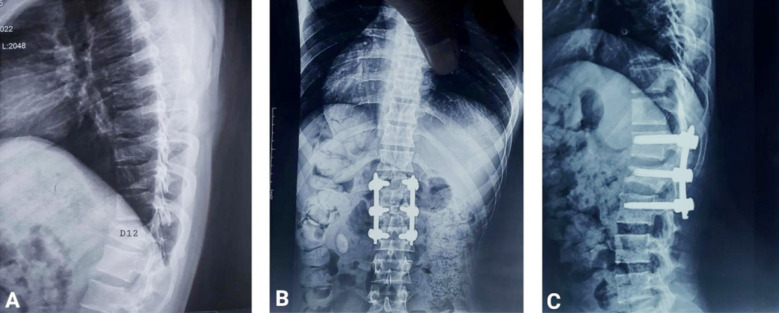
A: Preoperative X-ray dorsal spine lateral view showing D12 fracture, B&C: Postoperative X-rays AP view and lateral views for SSPF.
